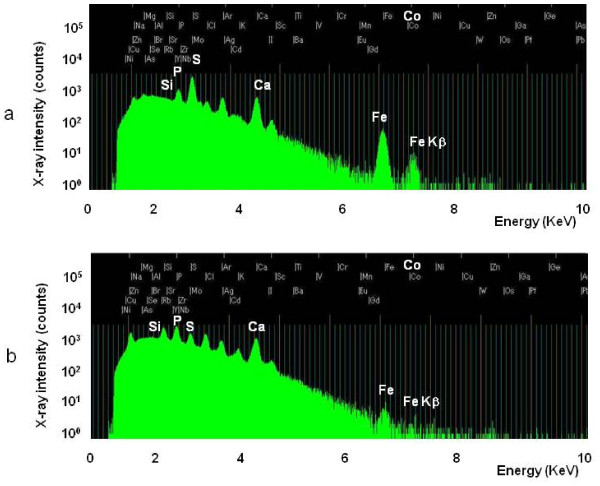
The X-ray peaks for each element obtained by in-air micro-PIXE analysis of alveolar macrophages from the surfactant-rich area in PAP and control lung. The microbeam was focused on a 54 μm × 61 μm area of the PAP lung tissue. Peaks display the characteristic X-ray signatures for each element, as shown by the counts (a). High peaks of S, Ca, and Fe were detected. The peak for Fe Kβ is near the peak of cobalt. The microbeam was focused on a 54 μm × 61 μm area of the control lung tissue (b). Peaks of S and Fe were lower than PAP lung tissue.
